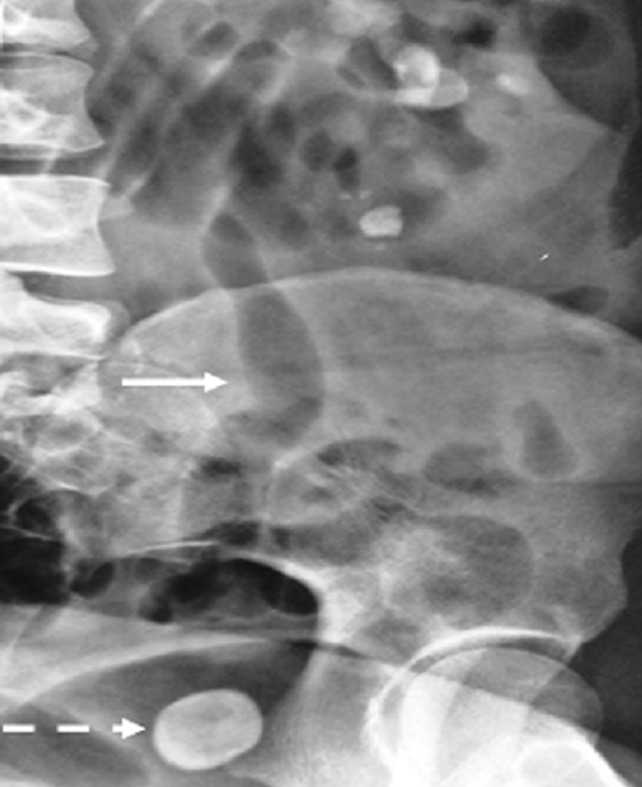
abdominal radiography of emphysematous pyelonephritis complicating ureterocele; full arrow: airy light in the left kidney and ureter; dotted arrow: ureteral pelvic lithiasis complicating ureterocele
